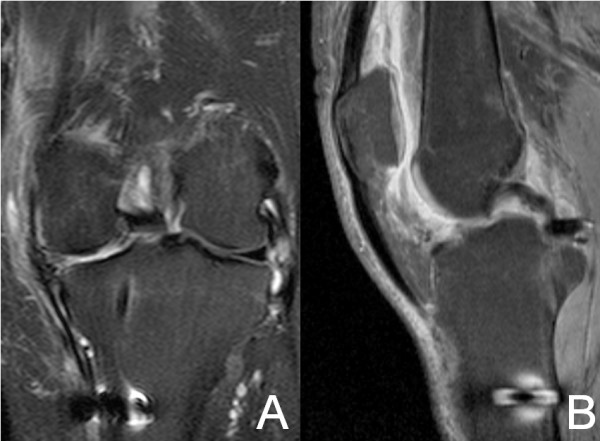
MRI in T1 sequences after semitendinosus graft implantation. In coronal plane (3A) the double semitendinosus tendon reaches the lateral margin of the lateral tibial plateau where CMI will be attached. In sagittal plane (3B) the posterior graft is adapted to the posterior tibial plateau near the PCL insertion.
